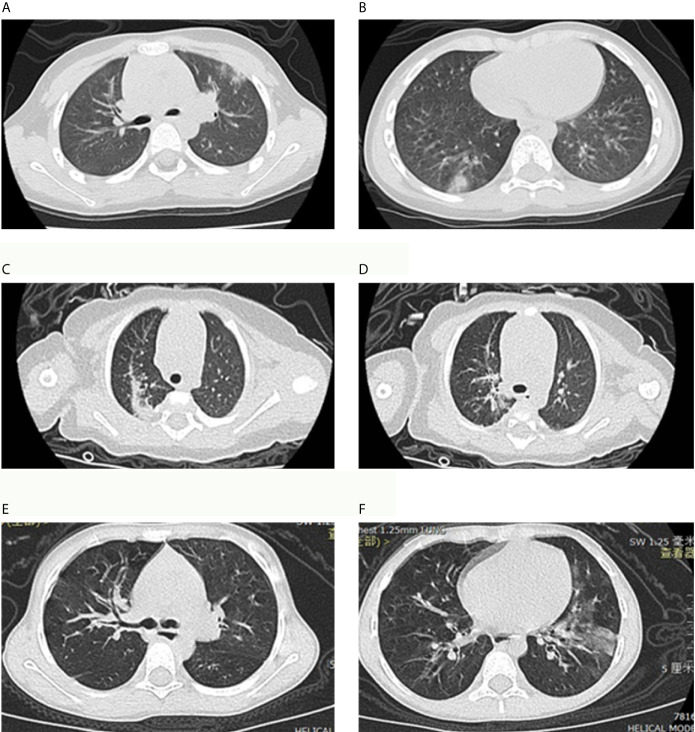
Chest computed tomography (CT) scans for COVID-19 pneumonia patient and Non-COVID-19 viral pneumonia patients. (A, B) showed ground-glass opacities were located in the right lower lobe of the lung and upper lobe of the left lung and located under the pleura in a patient with COVID-19 pneumonia. (C, D) showed multiple patchy shadows and consolidation in two lungs in a patient with respiratory syncytial virus (RSV) pneumonia. (E, F) showed thickened tracheobronchial wall in right lung and ground-glass opacities were located in the left lower lobe of the lung in a patient with influenza A (IFA) pneumonia.
